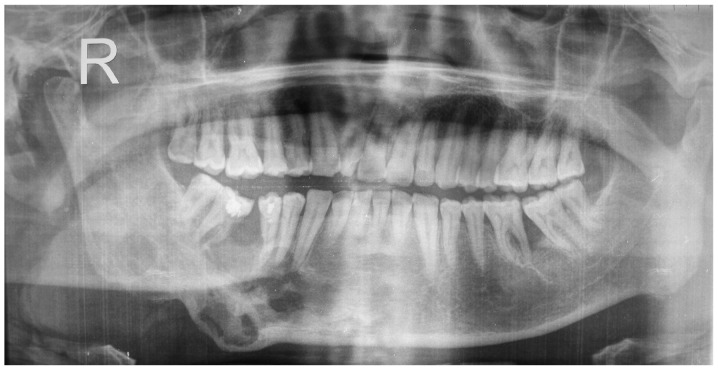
Second panoramic radiograph showing intralesional bone regeneration and malunion of the fractured mandible eight months later.
